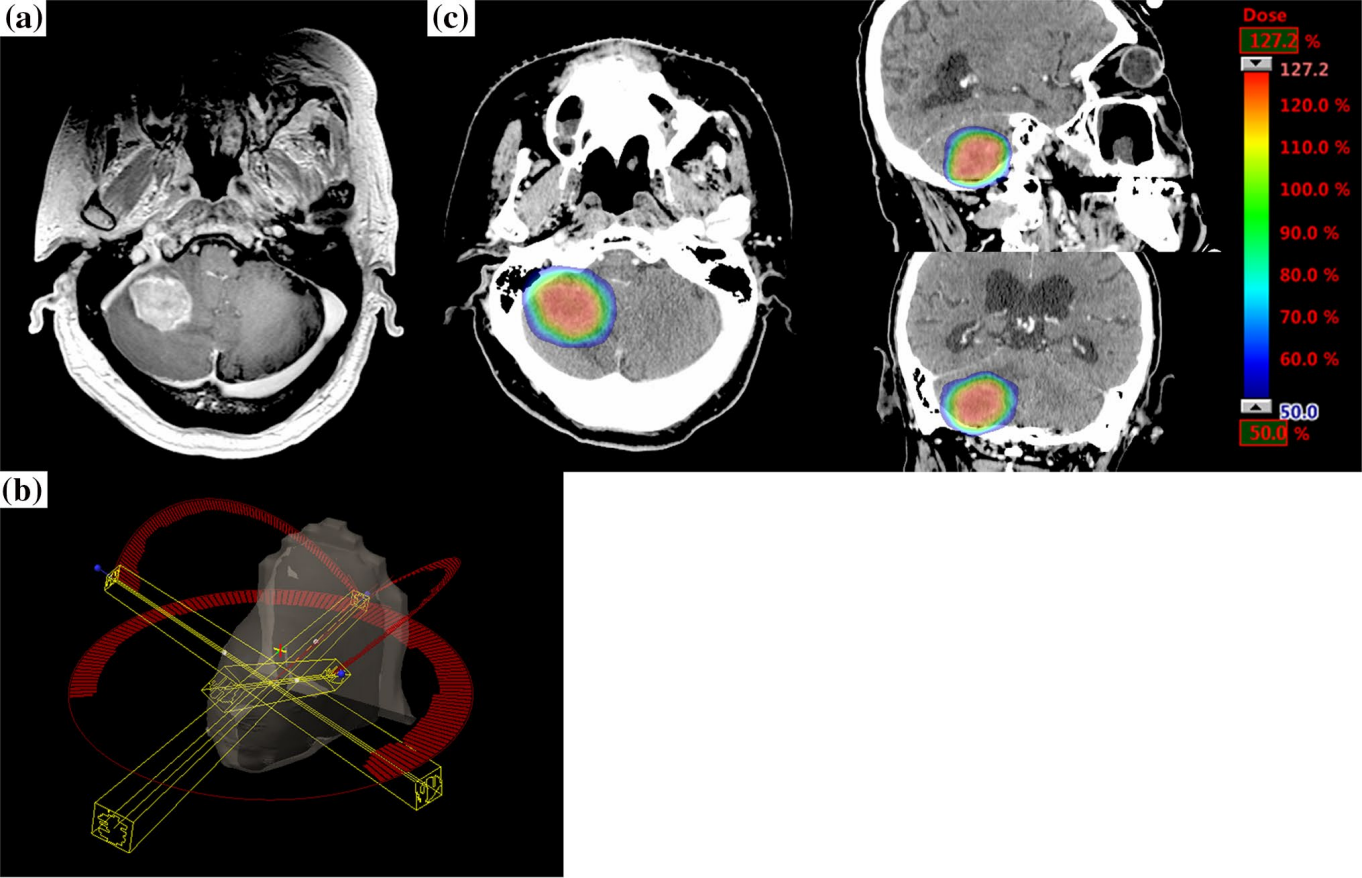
Treatment plan of brain metastasis by volumetric-modulated arc therapy (VMAT). a Axial: T1-weighted Magnetic resonance imaging (MRI) with gadolinium (Gd) before stereotactic radiotherapy (SRT). Gross tumor volume (GTV) was defined as the abnormality on the T1-weighted MRI with Gd. Planning target volume was generated by adding a 1-mm margin from GTV. b Axial coplanar and two non-coplanar arcs of VMAT. c Axial, Sagittal, Coronal: Dose distribution of SRT using VMAT
